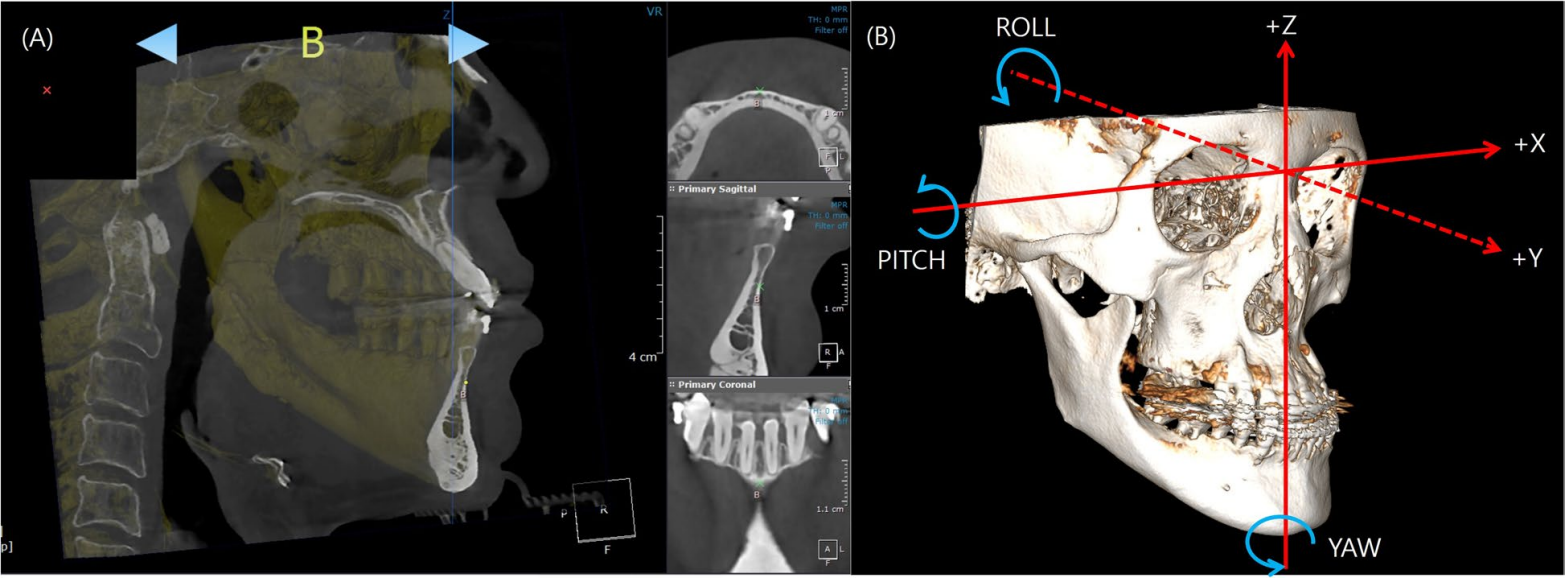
Alignment of the FH plane with the bilateral orbitale and pogonion points. The zero-point was set as Nasion. (A): Marking the B point on the 3D CT. (B): Definition of the x, y, and z axes, as well as yaw, pitch, and roll on the 3D CT. X-axis: ( +) left, (-) right; Y-axis: ( +) anterior, (-) posterior; Z-axis: ( +) superior, (-) inferior
